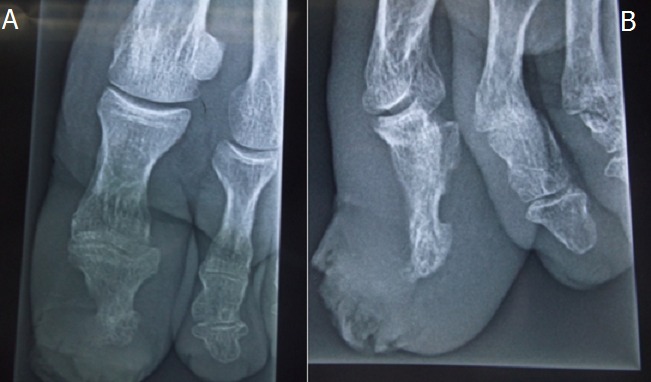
A) radiographie de face de l'orteil; B) radiographie de profil de l'orteil
